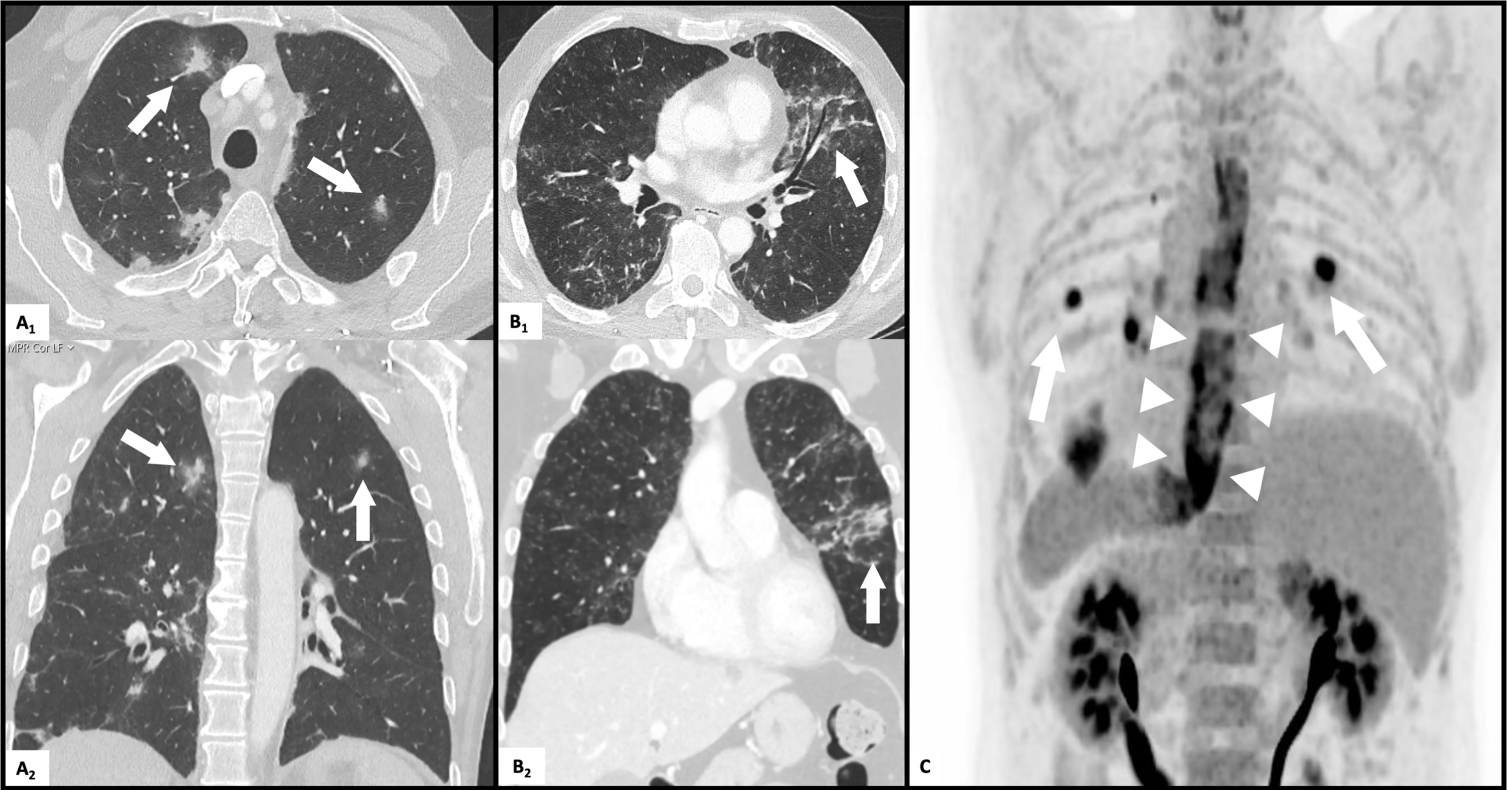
Pulmonary and esophageal affection on CT-scans. (A) multifocal nodular pulmonary infiltrates (arrows) on transverse (A_1_) and coronal (A_2_) high resolution CT scan. (B) diffuse pulmonary infiltrates (arrows) on transverse (B_1_) and coronal (B_2_) high resolution CT scan. (C) FDG-PET-CT scan: diffuse esophageal (arrowheads) tracer uptake and multiple pulmonary nodules (arrows).
